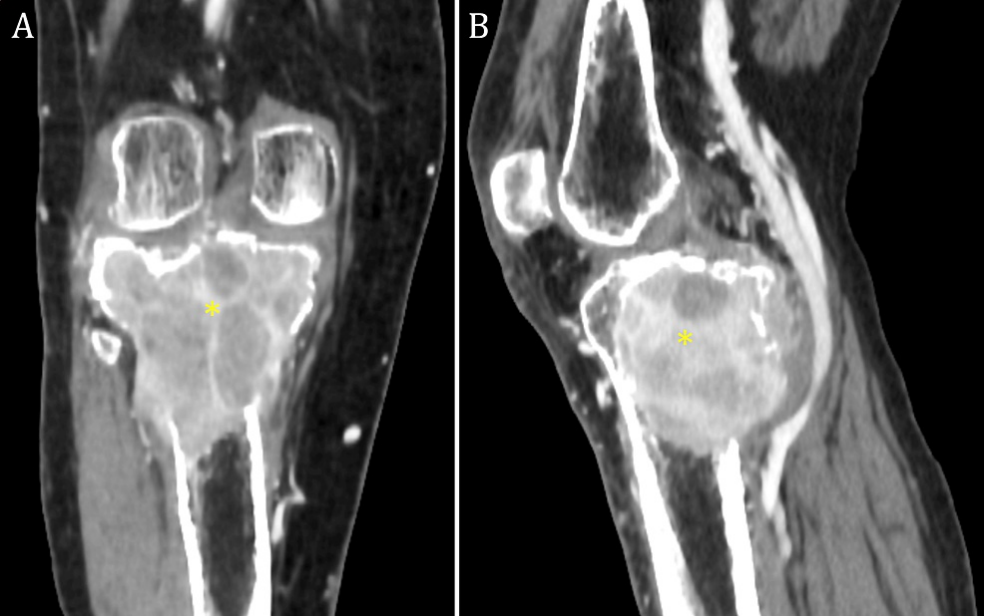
Preoperative contrast-enhanced computed tomography of the right knee: multiplanar reconstructions in the (A) coronal and (B) sagittal planes. Large lesion (*) in the proximal tibial epiphysis expanding past the cortical bone with a heterogeneous appearance, including necrotic and contrast-enhancing solid areas.
